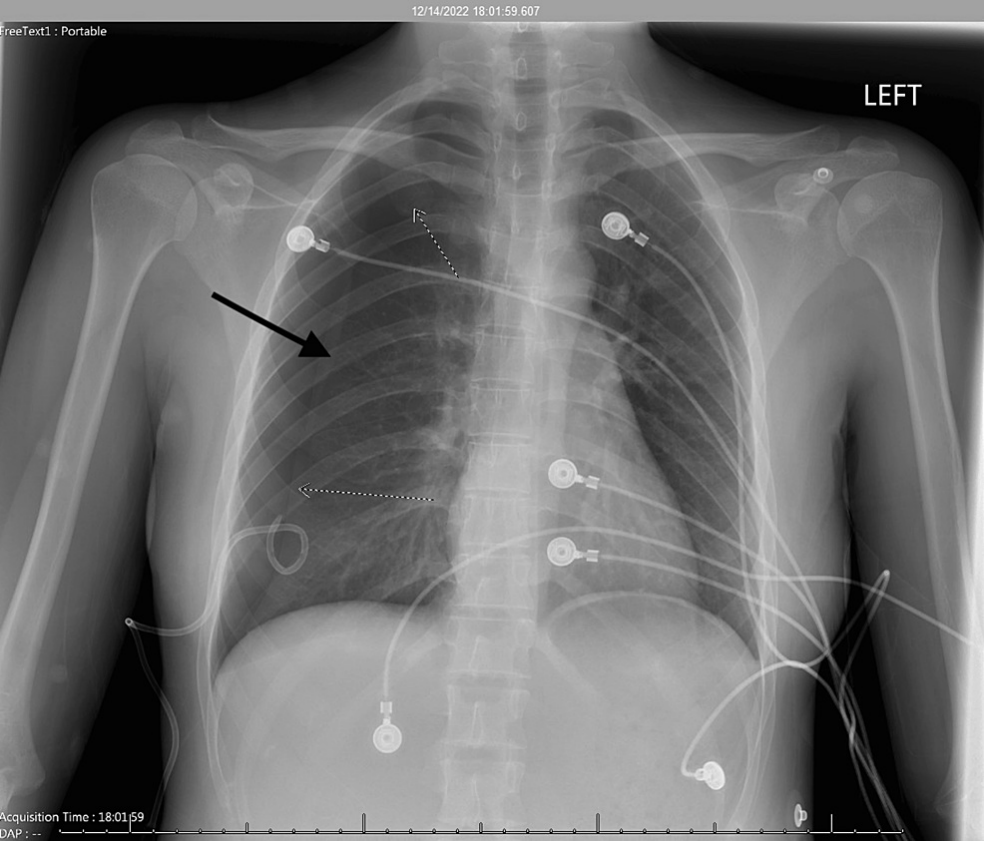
Chest x-ray #2 Impression: Pigtail catheter over the right lower lung. There has been interval development of a moderate to large right pneumothorax measuring approximately 50%, which has developed since the prior examination performed earlier the same day.  Mediastinal shift to the left. The lungs are otherwise clear. No left pneumothorax. No pleural effusion.
